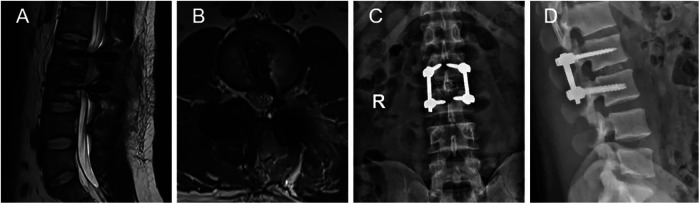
Postoperative radiographic images (A–D). (A,B) MRI revealed that the extruded lumbar intervertebral disc was removed. (C,D) Plain radiographs showed interbody fusion with posterior instrumentation.
